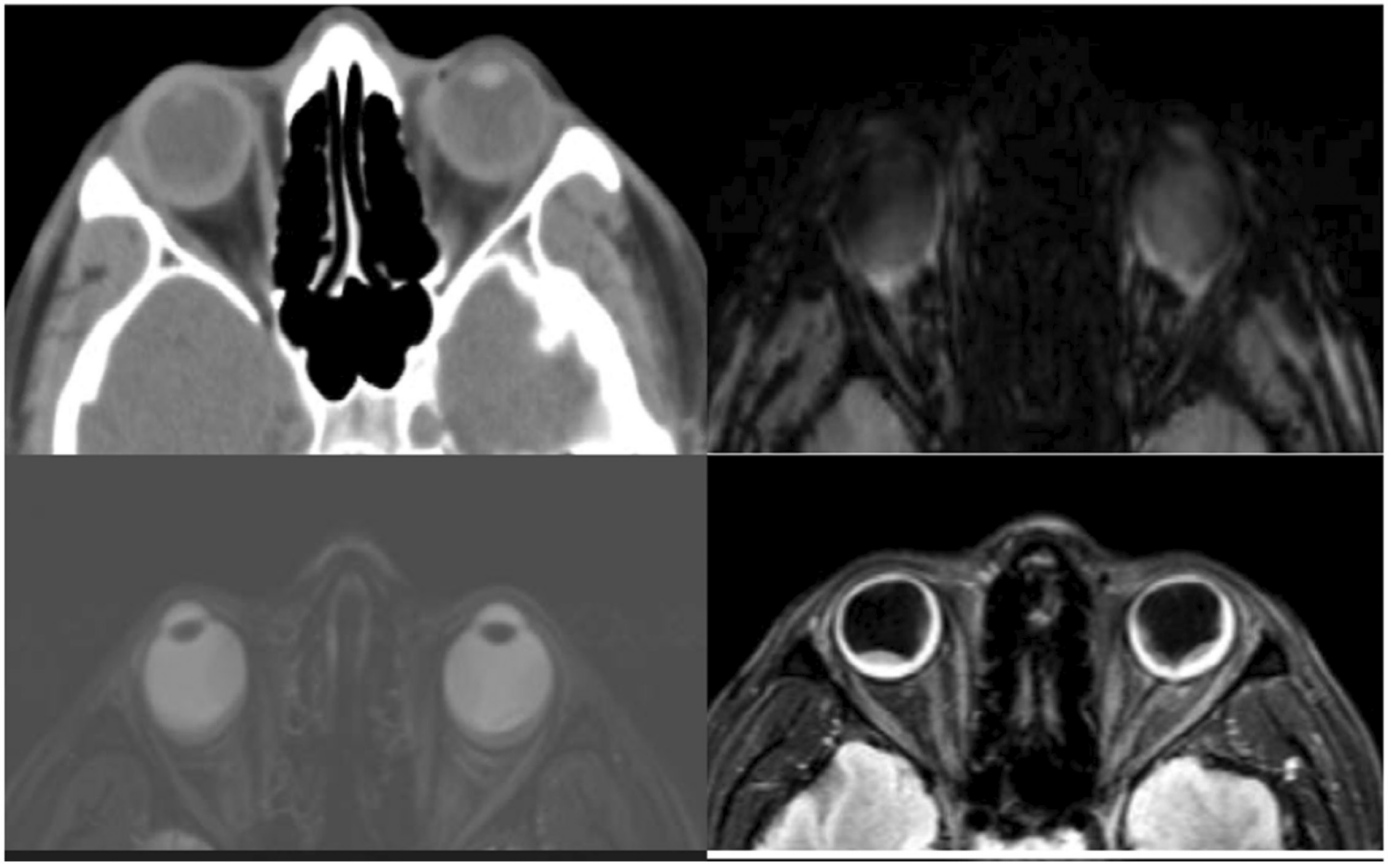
CT brain without contrast and sequential axial T1‐weighted MRI brain with and without gadolinium contrast demonstrating bilateral retinal detachment.
